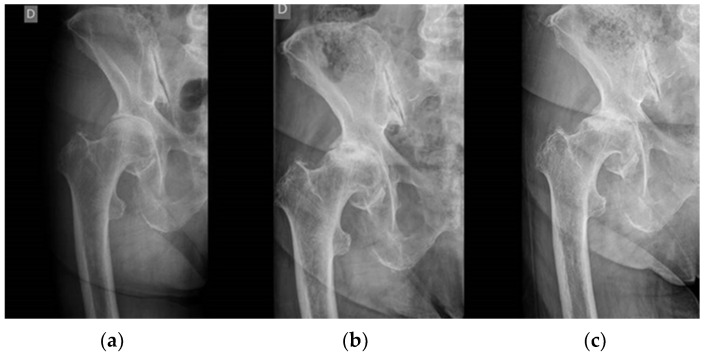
Radiological evolution of one of our patients 6 months after the onset of the first symptoms. (a), X-ray of the hip at the beginning of the symptoms; (b), radiographic study at 3 months and (c) at 6 months after the onset of symptoms. We can appreciate the rapid destructive evolution of the femoral head.
